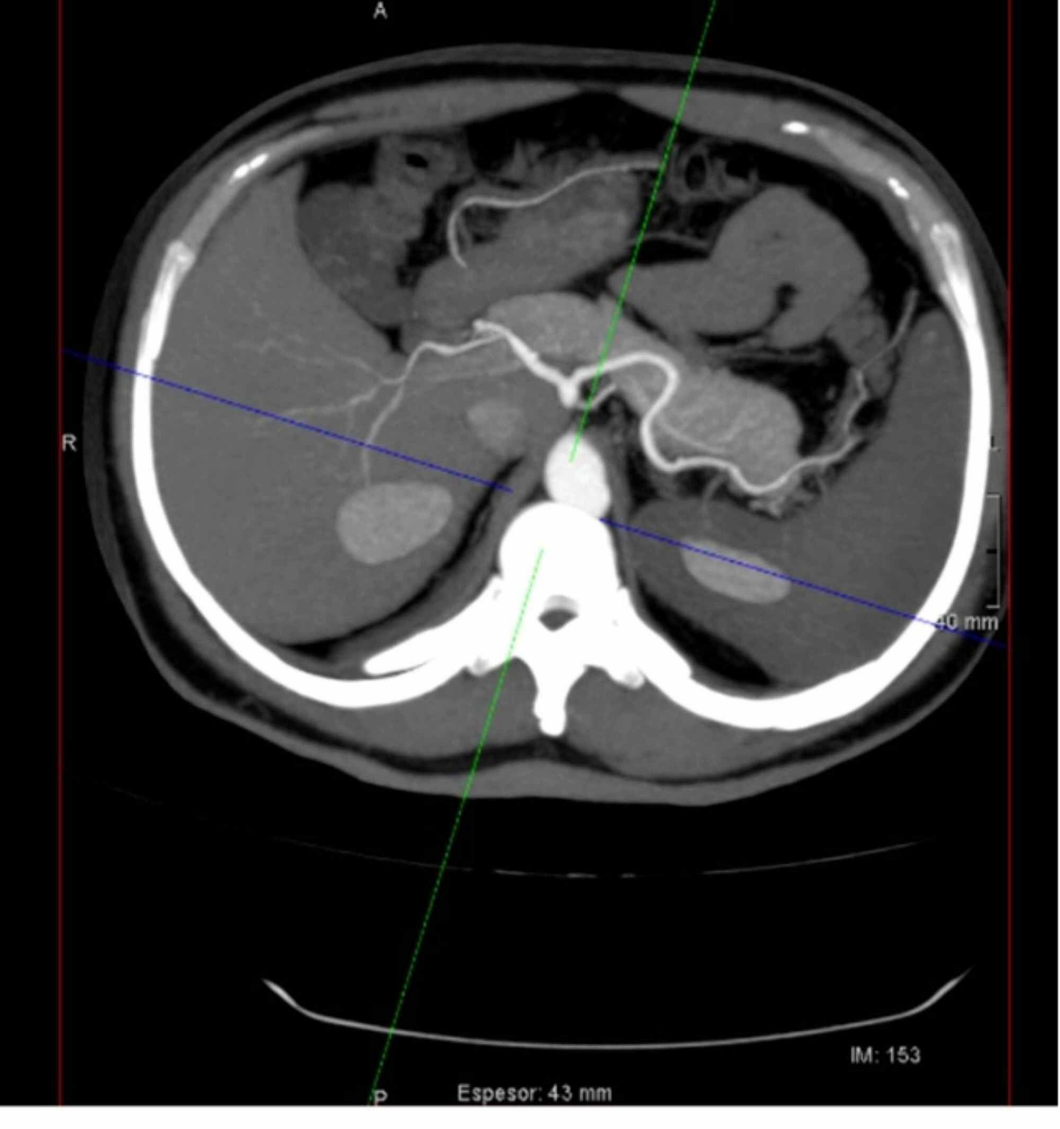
Axial contrast-enhanced CT of the abdomen Axial contrast-enhanced CT of the abdomen showing splenomegaly and lack of spleen enhancement suggesting splenic infarct.
